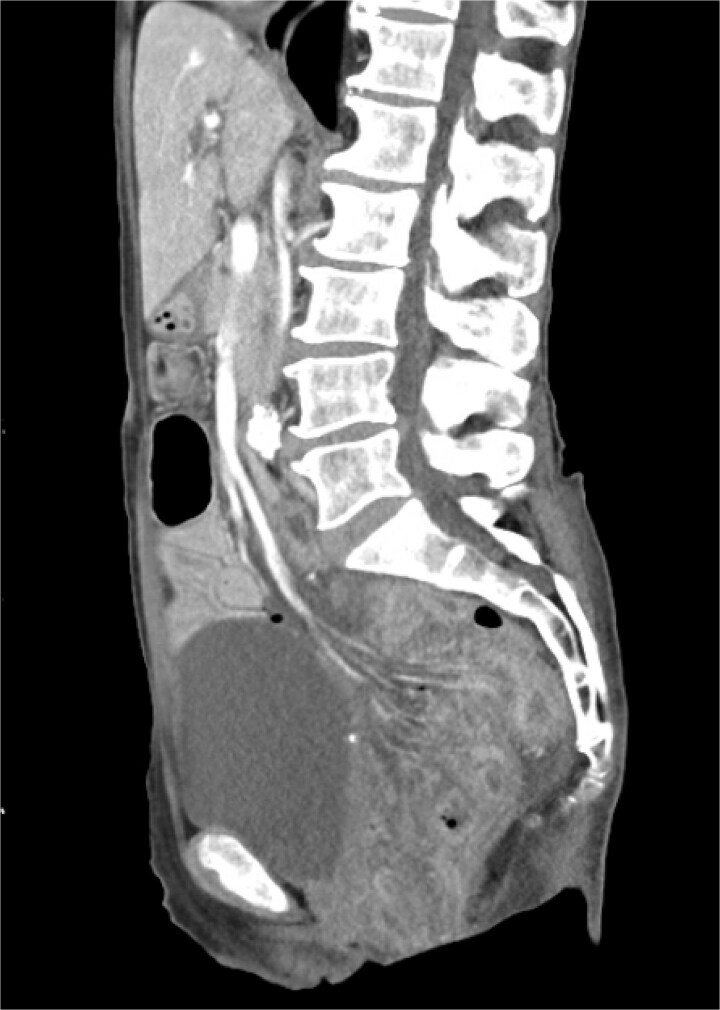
Sagittal view of contrast enhanced CT showing small bowel prolapse into the pelvis.
